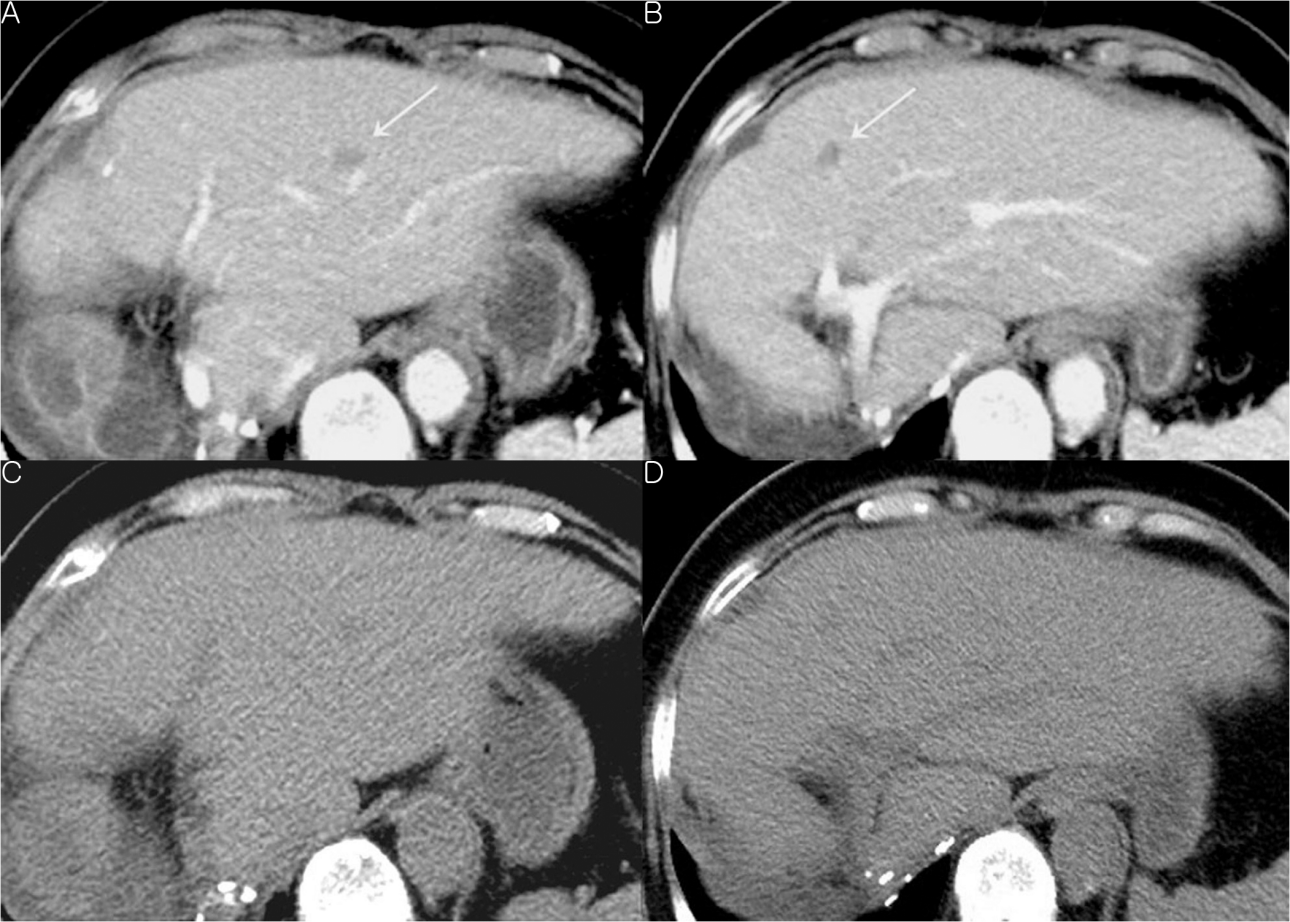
Seventy-year-old woman who underwent right hemicolectomy and right hemihepatectomy because transverse colon cancer with liver metastasis. (A, B) On follow-up CT, two newly developed metastases are seen in the remaining left lobe of the liver (arrows). (c, d) On non-enhanced CT images, low attenuating lesions were suspected at the same locations as those on contrast-enhanced CT, but neither of the reviewers could detect these lesions.
